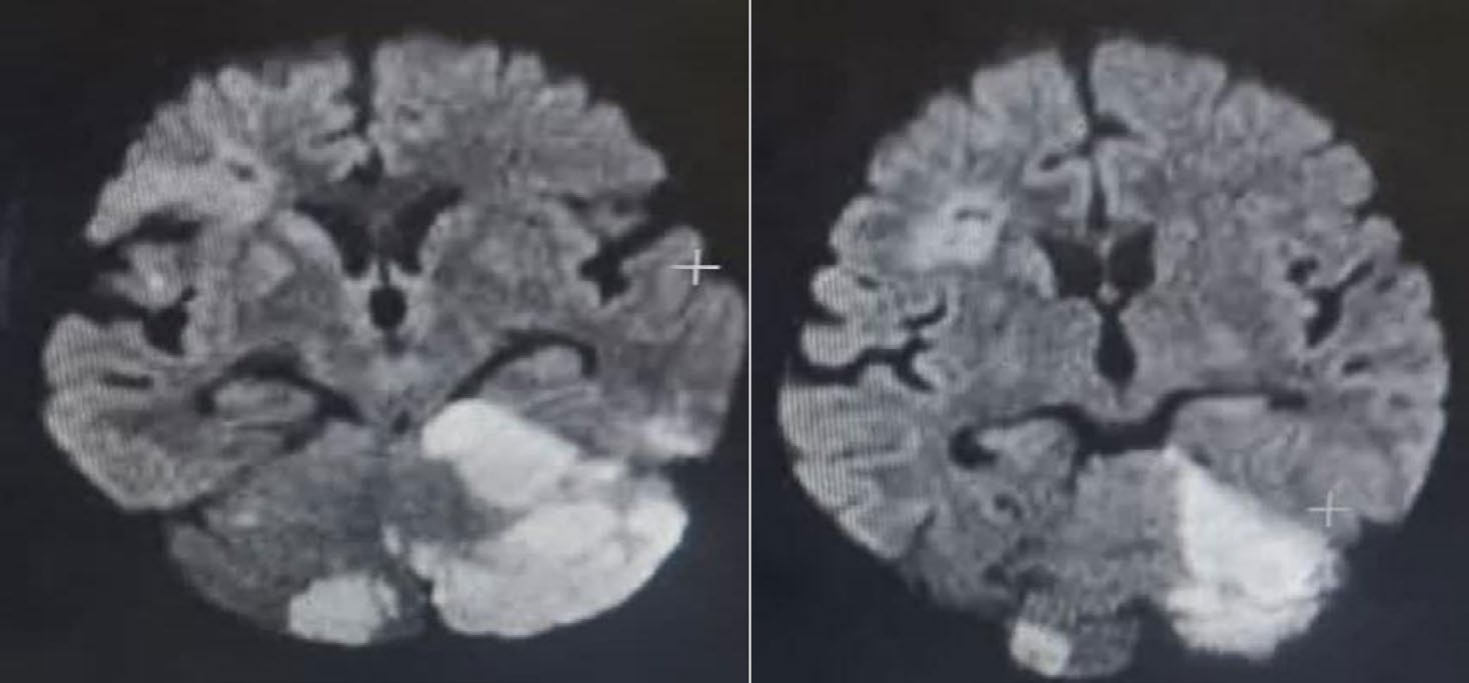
Contrast‐enhanced abdominal CT scan of the liver demonstrating a large, mass‐like lesion (50 × 45 mm) in the right hepatic lobe (arrow), with imaging characteristics highly suggestive of hepatocellular carcinoma.
